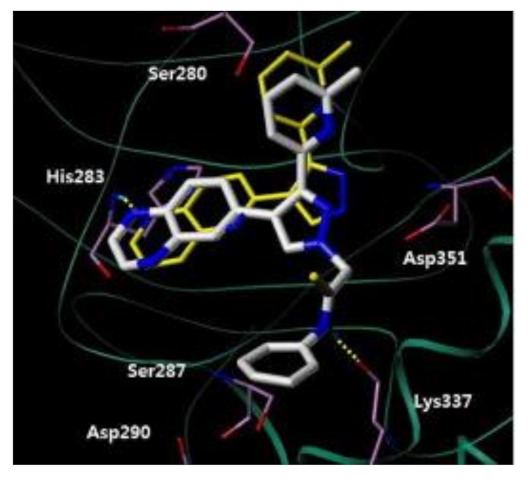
Binding pose of compound 78 in the active site of ALK5, superimposed over the X-ray pose of 1,5-naphthyrine inhibitor (yellow carbon). Yellow dotted lines indicate hydrogen bonding interactions (<2.5 Å) [90].
